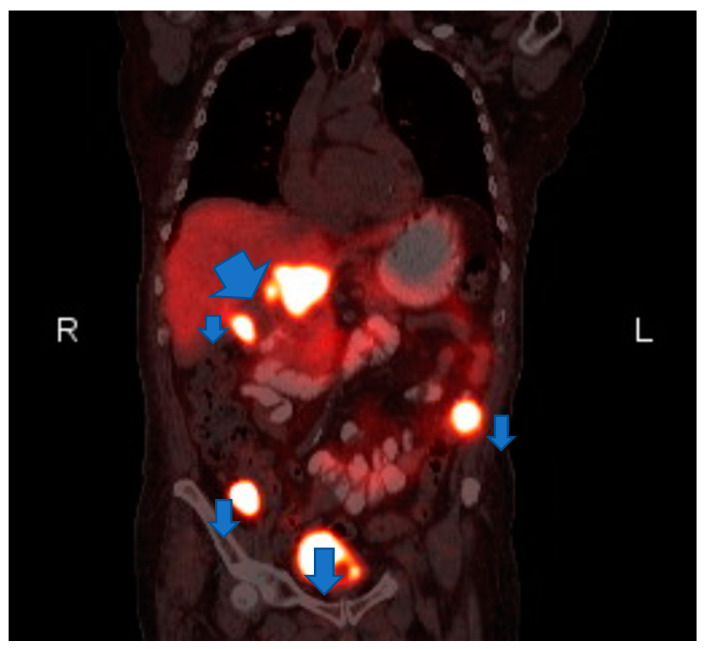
Coronal Postitron Emission Tomography/Computed Tomography (PET/CT). gallium-68 DOTA-DPhe1, Tyr3-octreotate (^68^Ga)Ga-DOTA-TATE Abnormal gallium dotatate accumulation in the right adrenalectomy bed, upper abdominal lymph nodes and two mesenteric nodules.
